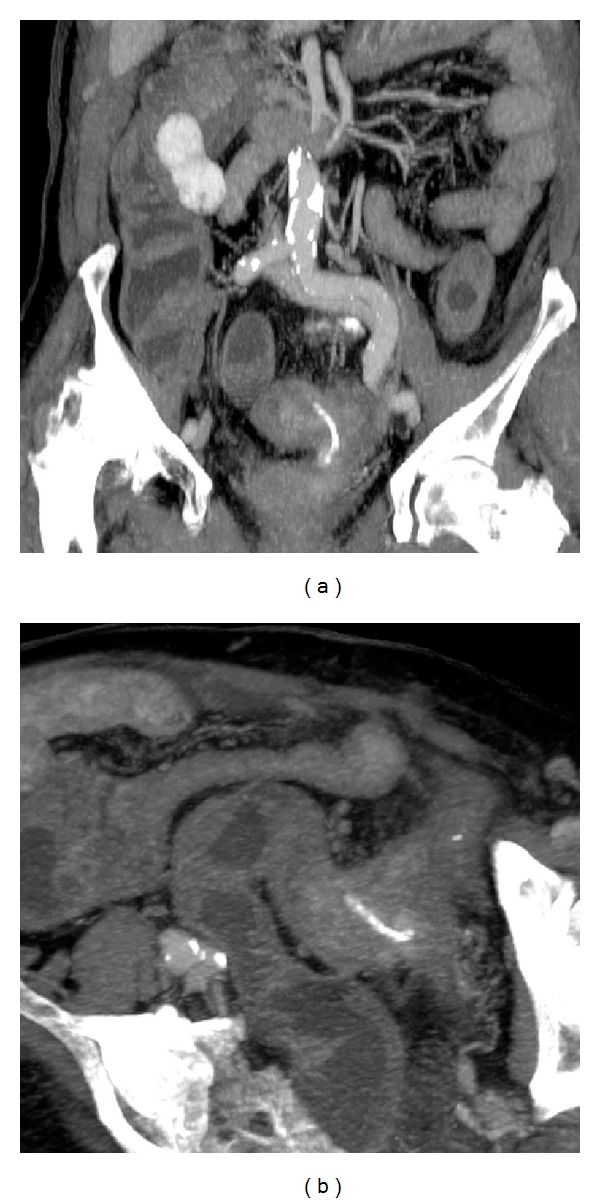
CT MPR images after contrast medium administration in portal (a) and delayed phase (b) showing the curvilinear sharp-pointed foreign body in the sigma and the contrast-enhancement within the inflammatory pseudotumoral mass at the FB tip.
